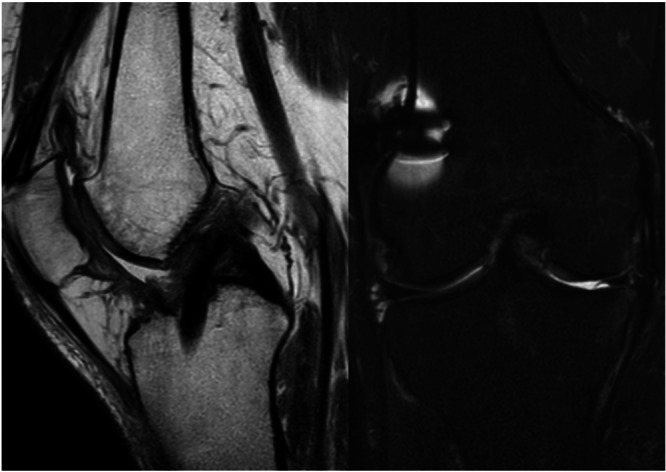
Sagittal (left) and coronal (right) MRI of a 63‐year‐old patients 3 years after ACL reconstruction with hamstring ‘Over‐the‐Top’ and associated 'Lateral‐Extra‐Articular tenodesis' and medial meniscus bucket‐handle repair. ACL, anterior cruciate ligament; MRI, magnetic resonance imaging.
